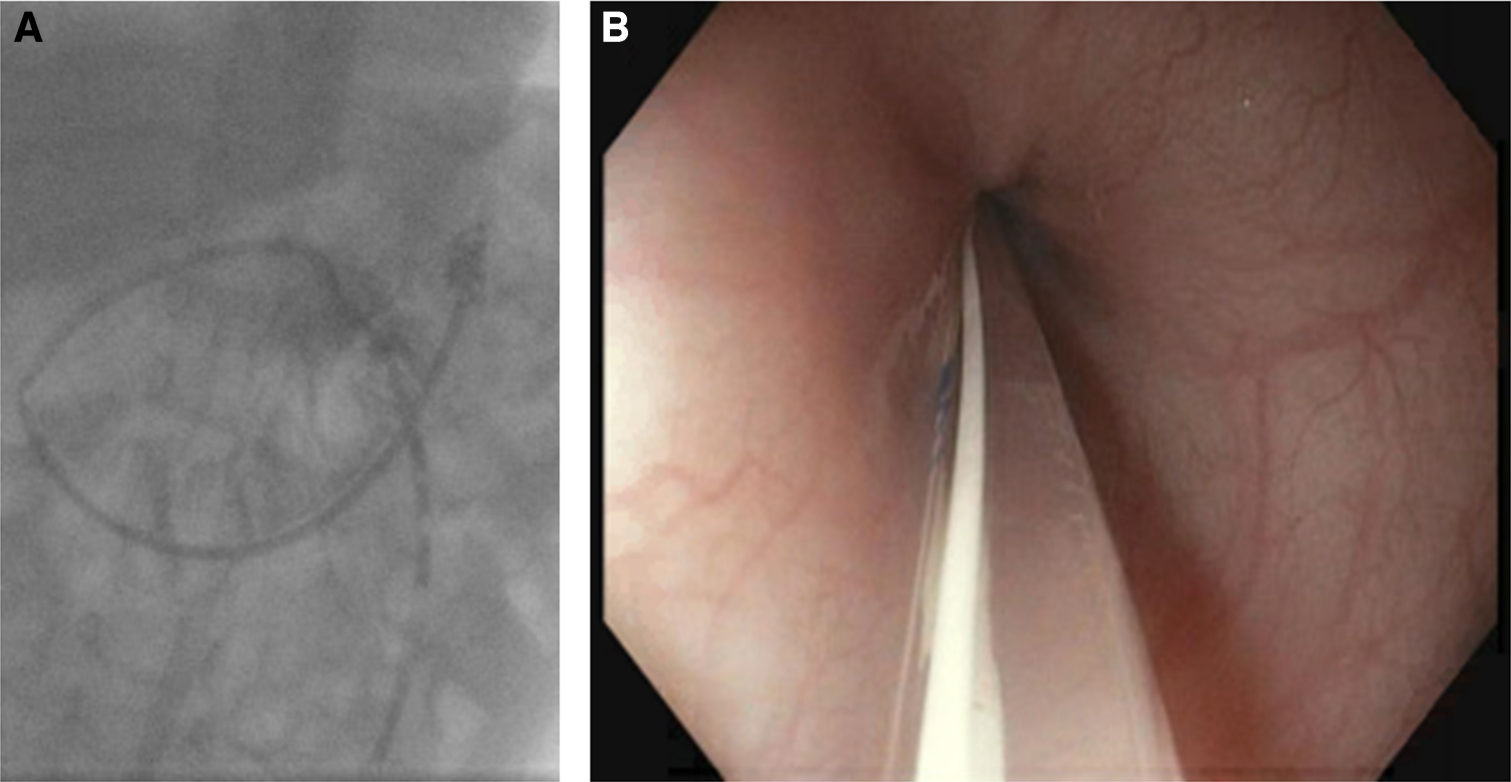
(A) A J-PEG is shown using an x-ray. (B) A J-PEG in front of the pylorus, recorded during endoscopic placement.
